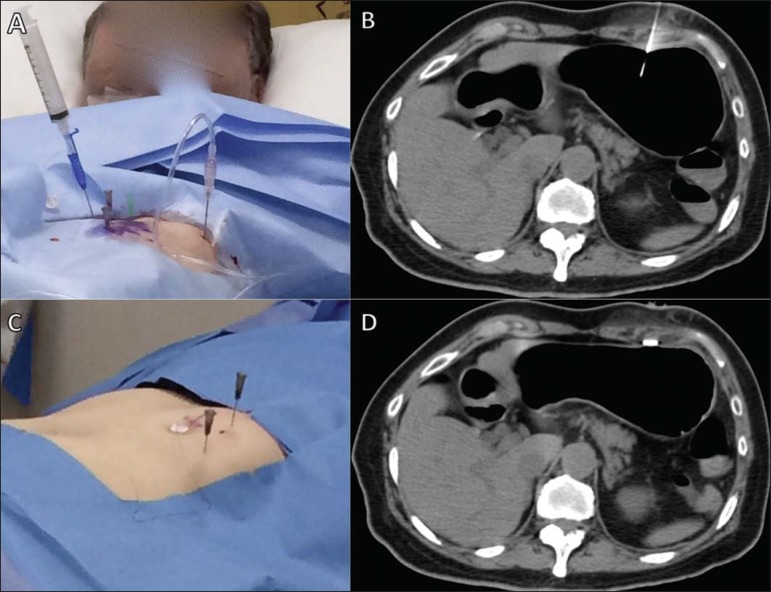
Fixation of the gastric wall for CT-guided percutaneous
